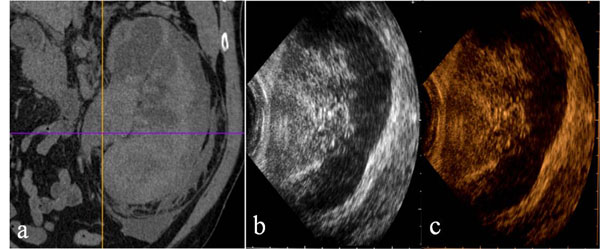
Kidney. Contrast-enhanced CT in venous phase (a), US-B-mode (b) and CEUS (c) in a 72 year-old female patient with blunt abdominal trauma. CT shows a renal subcapsular haematoma (a) recocognizable by US B-Mode examination (b).and CEUS corresponding to that of CT.
